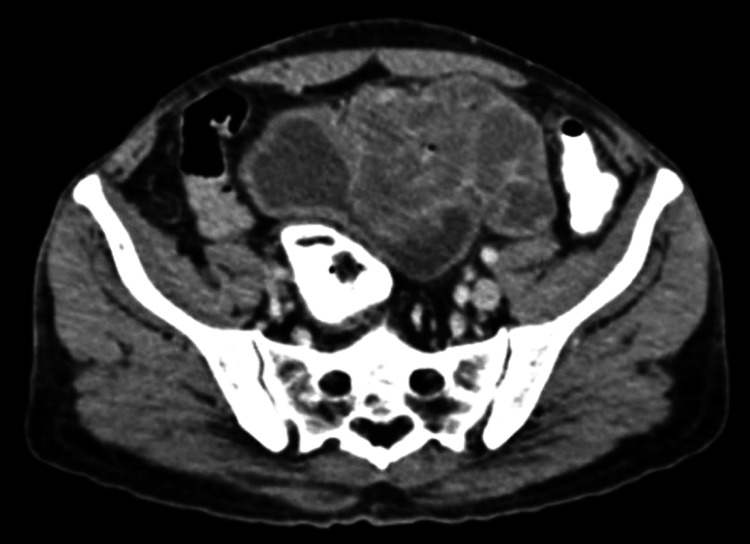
Contrast-enhanced computed tomography (CECT) image showing the sigmoid colon mass extending into the urinary bladder
